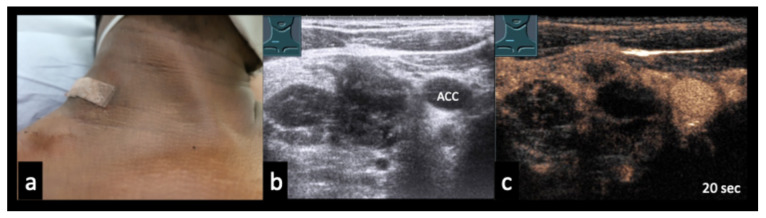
30-year-old patient with confirmed cervical lymph node tuberculosis (a). (b) B-mode ultrasound shows an inhomogeneous conglomerate lymph node mass. (c): CEUS demonstrates partial absence of enhancement, consistent with necrotizing tuberculous lymphadenopathy. ACC = common carotid artery.
